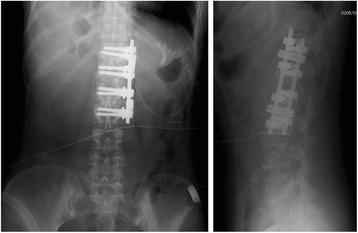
Postoperative radiographs. Postoperative anteroposterior (*left*) and lateral (*right*) radiographs of the spine show the anterior spinal fusion with instrumentation from T12 to L3
